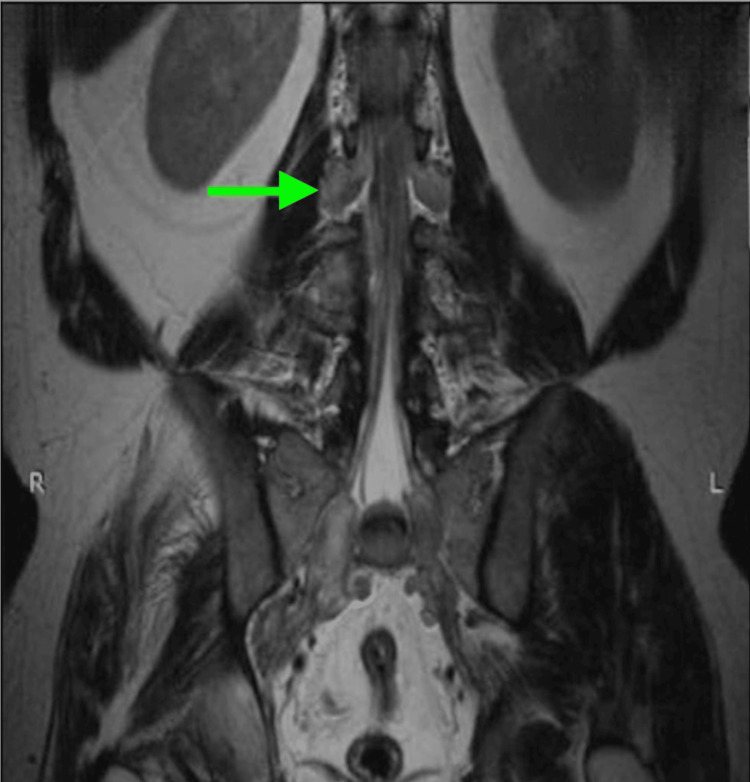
Coronal T2-weighted MRI MRI showing focal enlargement of a lumbar nerve root, consistent with chronic hypertrophic demyelinating neuropathy (green arrow).
